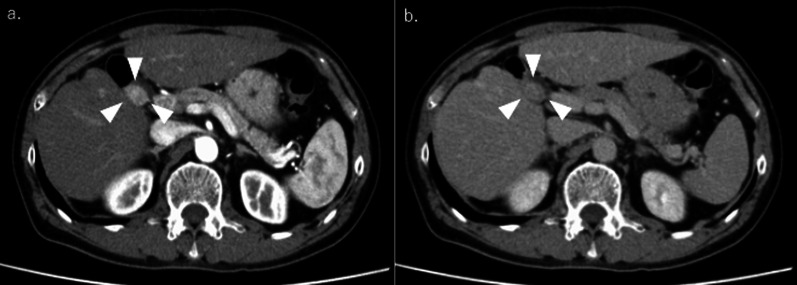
Contrast-enhanced CT. (a early phase, b delayed phase) showed a mass lesion of 2.5 cm in diameter at the hepatic hilum (white arrow)
